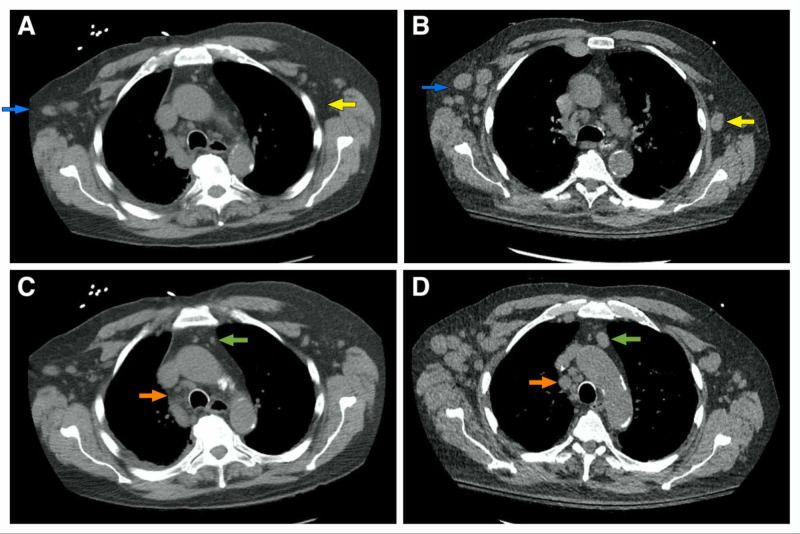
CT scan of the chest Increased bilateral axillary adenopathies (blue and yellow arrows) are smaller in the previous CT scan done 10 months prior (A) when compared to the new scan (B). Similarly, increased mediastinal adenopathies are evident (green and orange arrows) when comparing an old scan (C) with the newer study (D) CT: computed tomography
